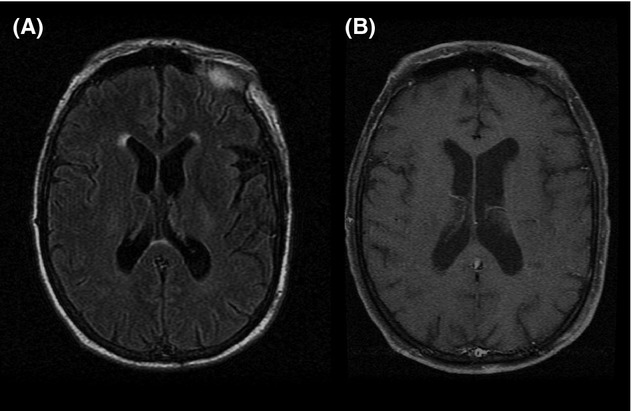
MRI images obtained on admission. (A) Axial FLAIR image that is unremarkable, without any significant hyperintensities. (B) Axial T1-weighted postcontrast image that is unremarkable, showing no abnormal areas of enhancement.
